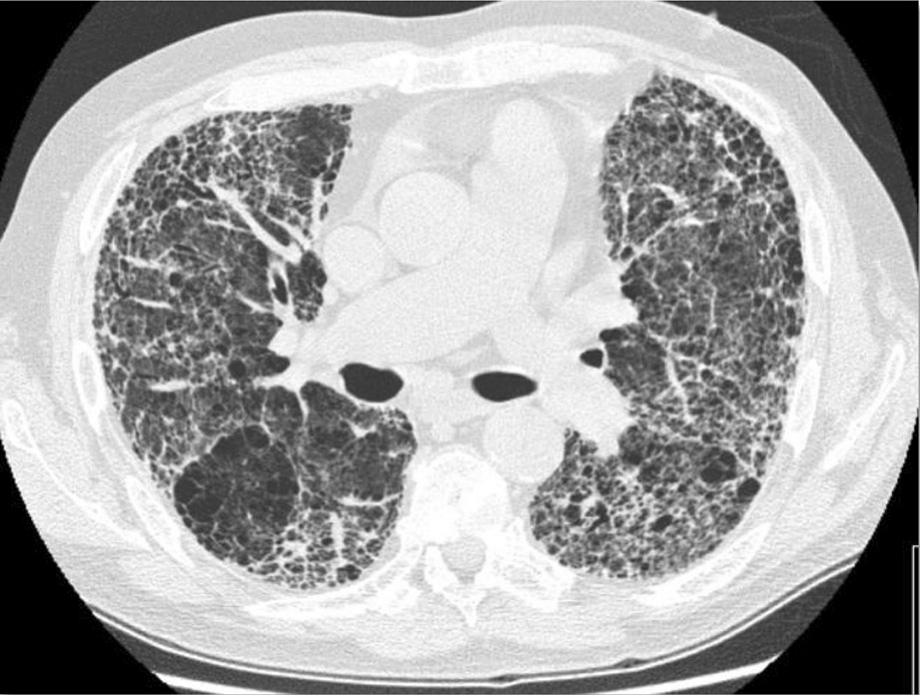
Case 1: CT scan of a retired 75-year-old man, ex-smoker, showing idiopathic pulmonary fibrosis. *CT* computed tomography
